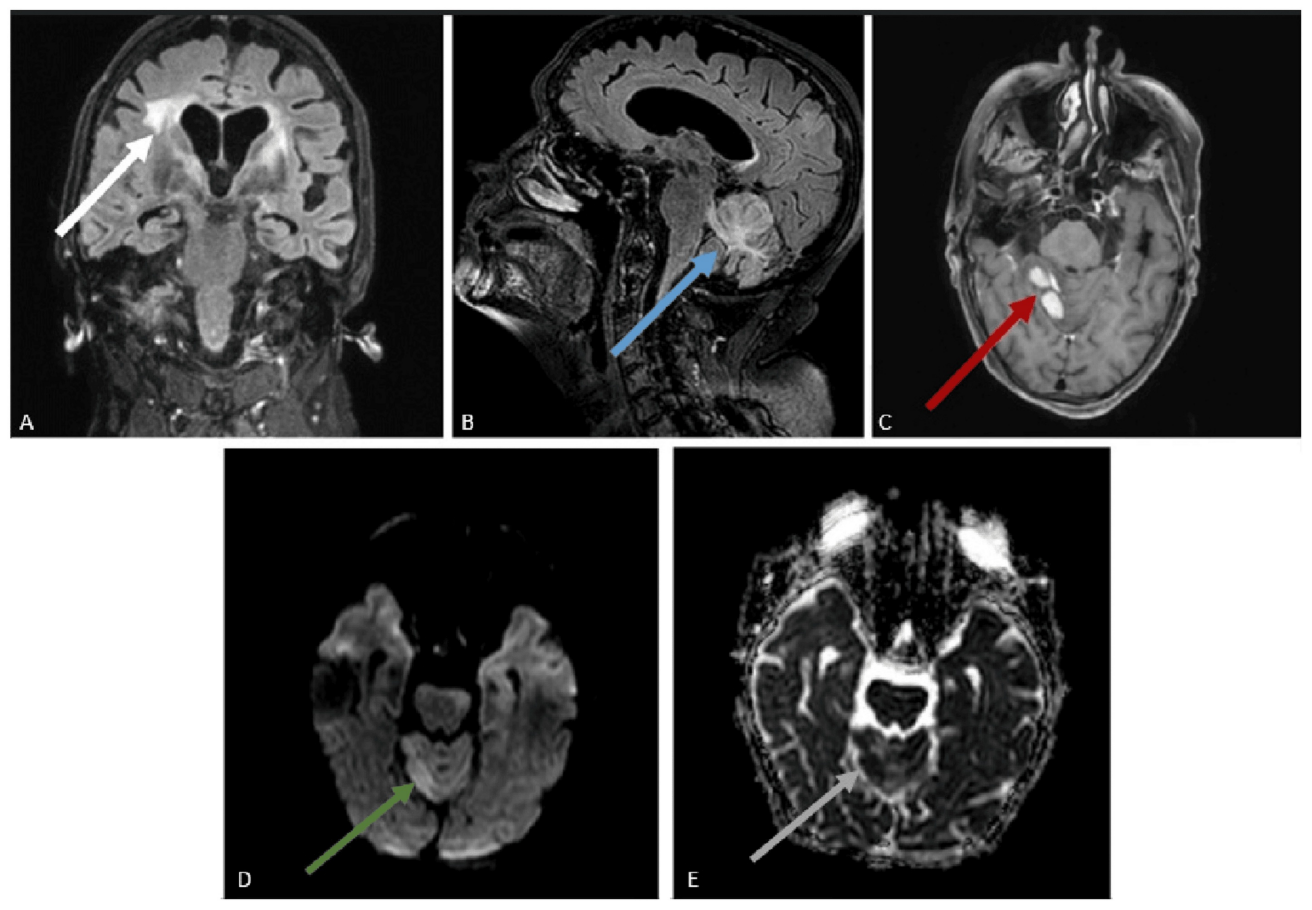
Brain MRI findings eight months after corticosteroid therapy: Coronal (A) and sagittal (B) FLAIR images show cortical and subcortical hyperintense lesions in the periventricular white matter (white arrow) and cerebellum (blue arrow). A T1-weighted sequence with gadolinium contrast (C) demonstrates a homogeneously enhancing cerebellar lesion (red arrow). Diffusion-weighted imaging (D) reveals restricted diffusion within the cerebellar lesion (green arrow), confirmed by corresponding low signal intensity on the apparent diffusion coefficient map (grey arrow) (E). MRI, magnetic resonance imaging; FLAIR, fluid-attenuated inversion recovery
